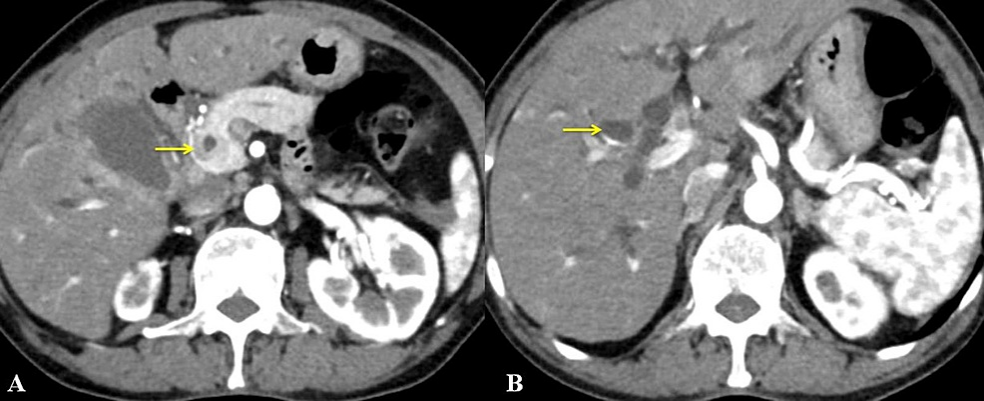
Computed tomography of the abdomen (axial view) showing; (A) well-defined circumferential wall thickening in the distal common bile duct (yellow arrow) and (B) dilated intrahepatic biliary radical (yellow arrow).
